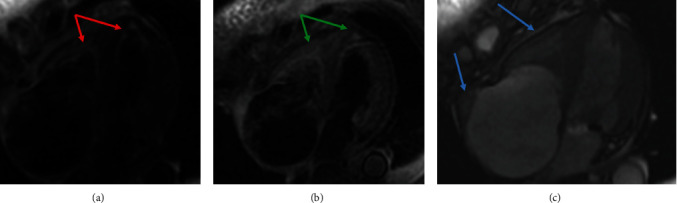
CMR. (a) Double inversion recovery (IR) T2-weighted axial imaging showing increased signal intensity of myocardium in the appendix of left and right ventricles consistent with myocardial edema (red arrows). (b) Tripe IR T1-weighted fat saturation axial image showing again signal intensity in the myocardium (green arrows). (c) Balanced steady state free precession (bSSFP) horizontal long axis still frame showing increase signaling with severe right atrial enlargement and possible thrombus (blue arrows).
